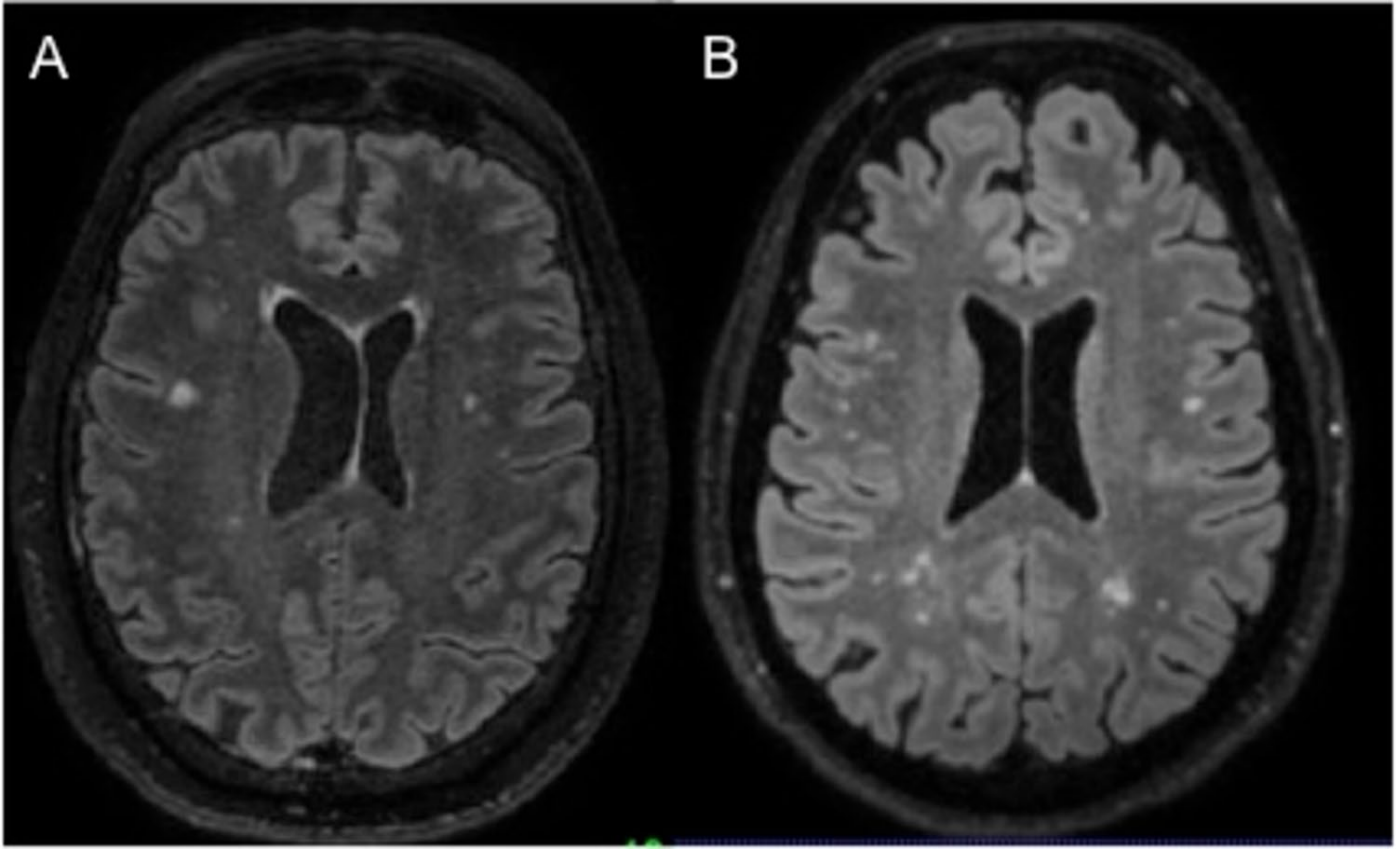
Atypical MRI lesions in patients with an alternative diagnosis. a Bilateral small juxtacortical frontal T2-hyperintese lesions, the larger one within the right inferior frontal gyrus (5 mm longitudinal axis) in a patient with anti-MOG encephalopathy. b Bilateral small juxtacortical, deep and periventricular frontal parietal and T2-hyperintese lesions, partially confluent within the posterior white matter in a patient with vascular encephalopathy
